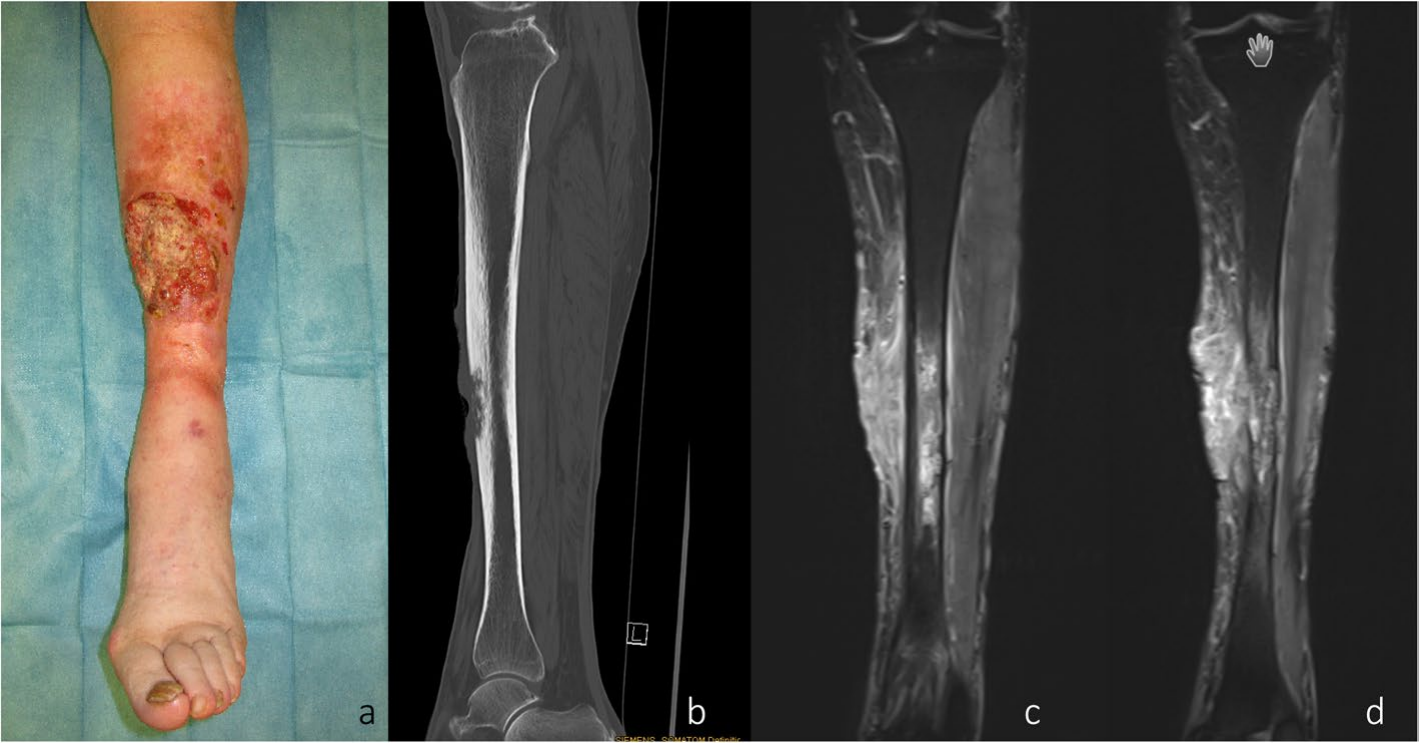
a Preoperative clinical photo. b Sagittal slice of preoperative CT scan (latero-lateral). c Coronal slice of preoperative MRI. d Coronal slice of preoperative MRI
